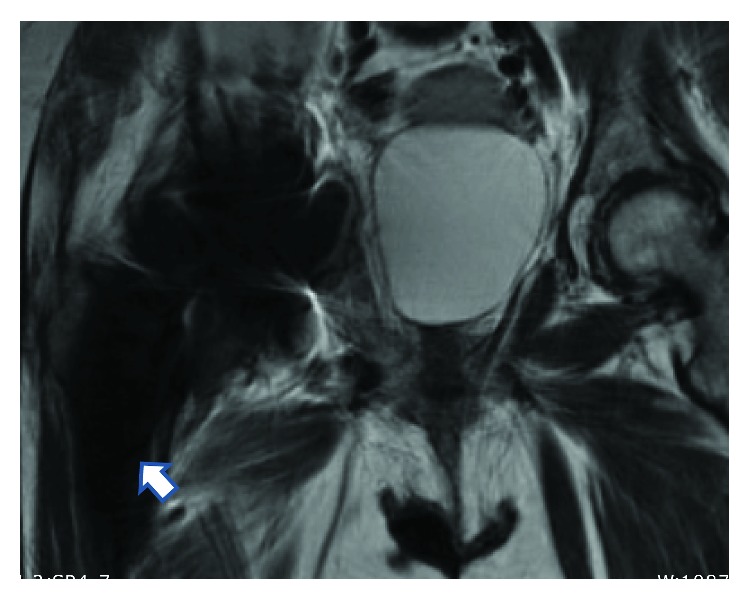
MRI right hip. Large air- and fluid-filled collection tracking along the iliopsoas bursa and psoas muscle (arrowhead).
